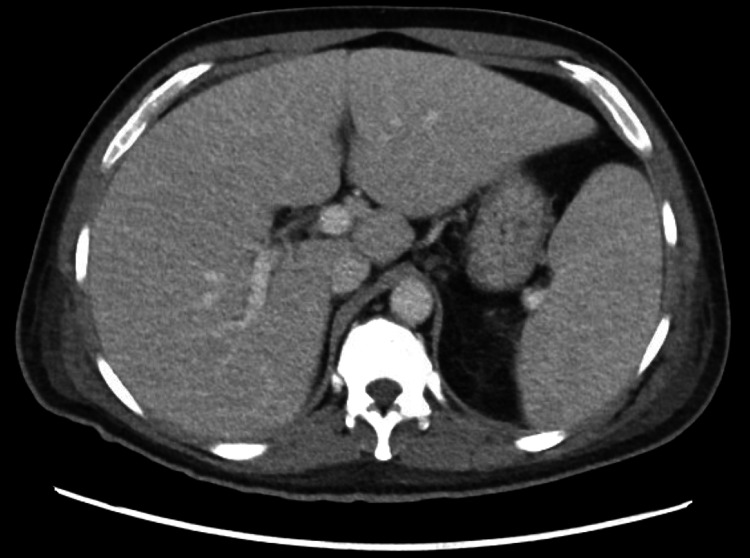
CT abdomen and pelvis demonstrating enlarged spleen (splenomegaly)
